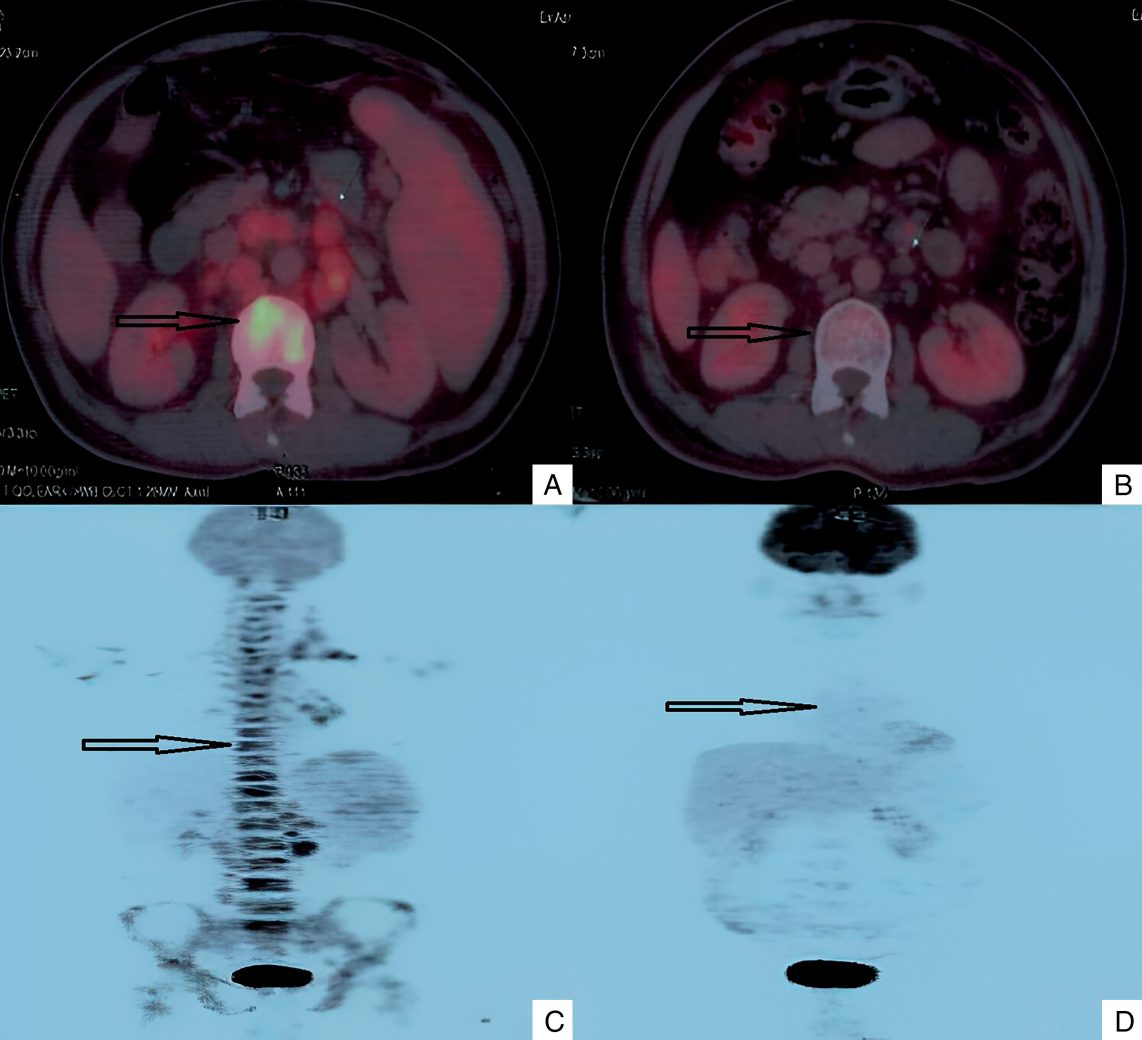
Pre (A) and post (B) treatment PET, and corresponding pre (C) and post (D) treatment CT images suggesting a significant response (arrow) to the therapy.
